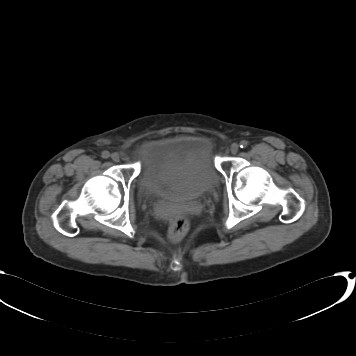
Three months after TUR, CT showed the tumor recurrence at the posterior wall and bladder neck and the border with urethral orifice was not clear.
